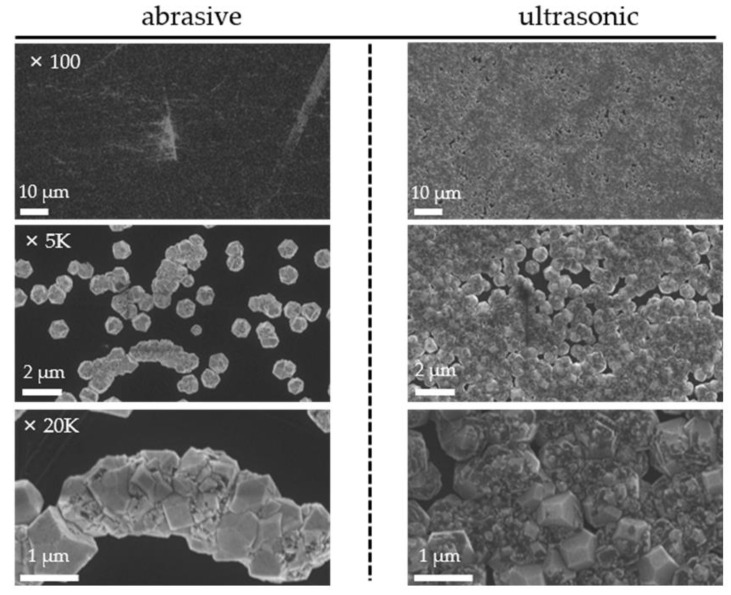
SEM images of the substrate surface after 6 h of deposition with substrates prepared via mechanical scratching and ultrasonic seeding methods at various magnifications.
